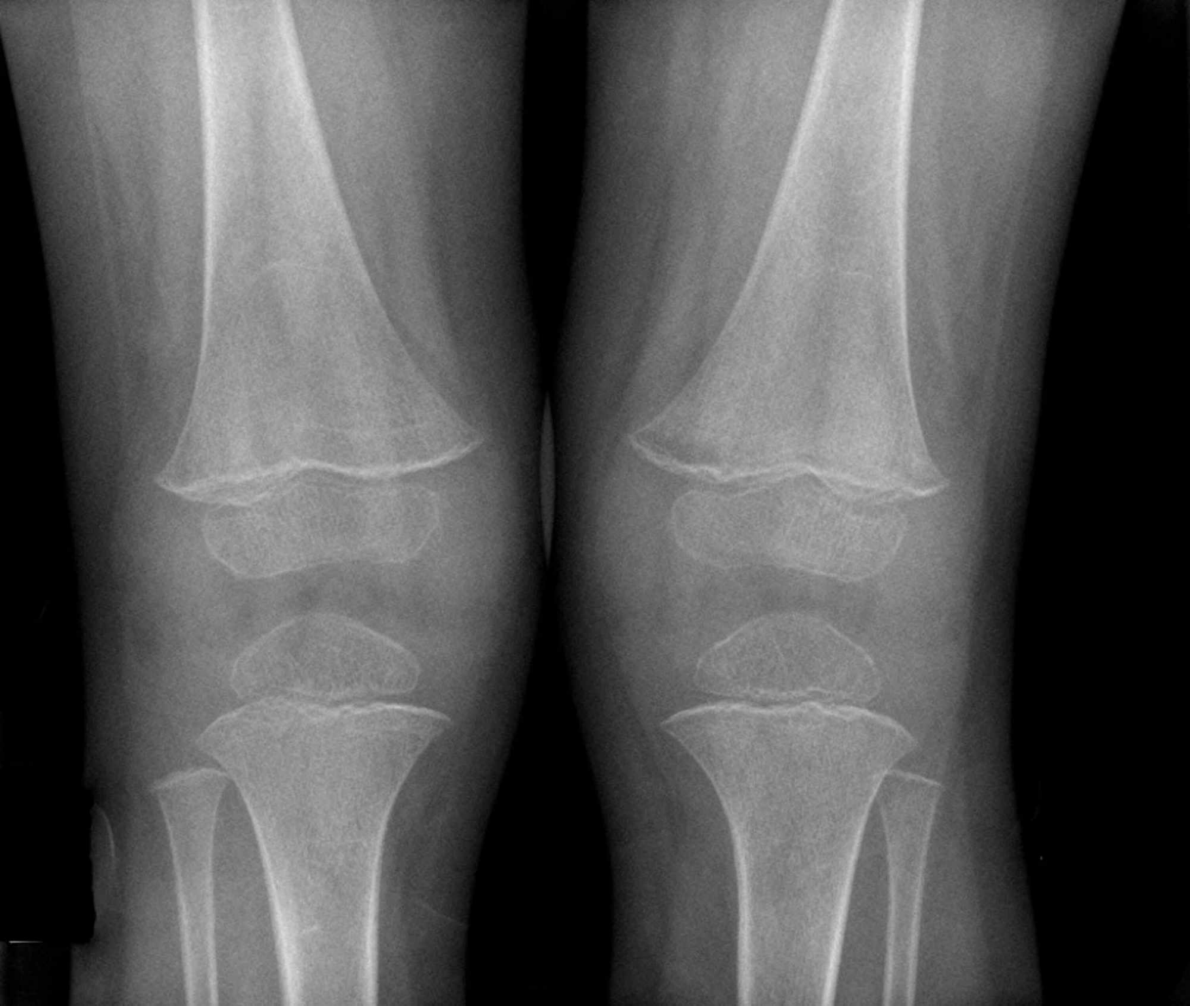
X-ray of both the knees from third presentation to hospital
